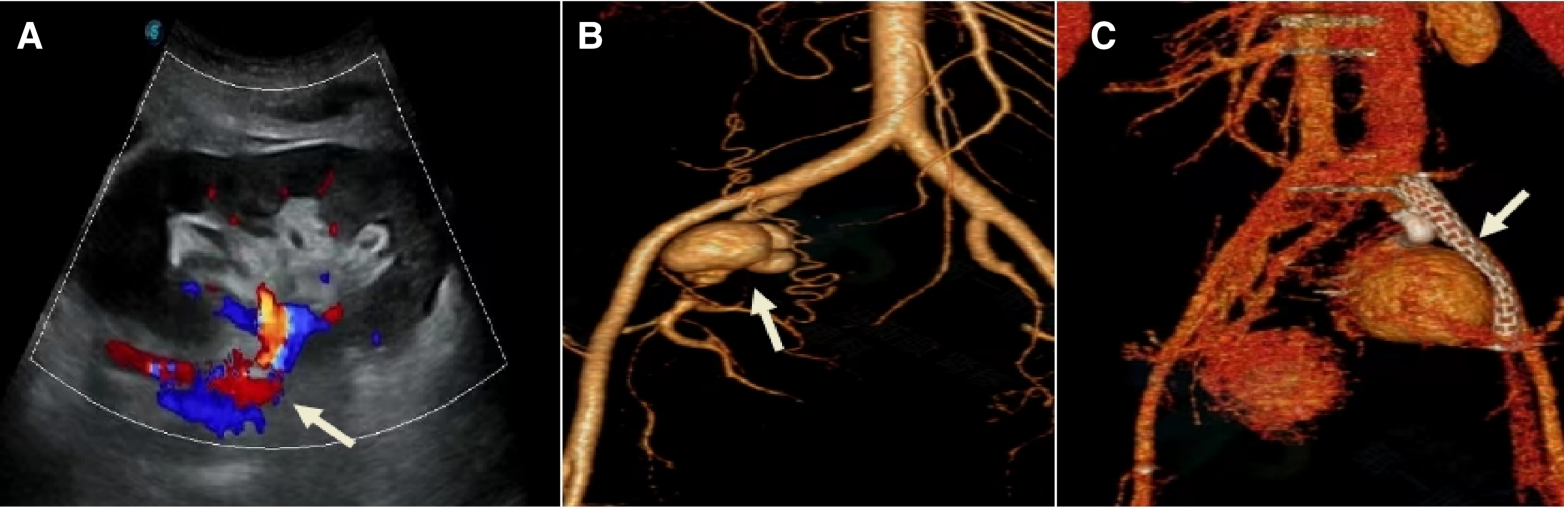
Treatment modality of the transplanted renal aneurysm. Patient 7, female, 34 years old, 2.5 months after renal transplantation, oliguria for 1 day, Scr 354 umol/l. (A) Ultrasound of the transplanted renal before admission: Poor perfusion of the renal, with an anechoic area behind the right external iliac artery, about 32 mm × 36 mm in size, connected to the renal artery, with a channel opening of about 6 mm in width. (B) DSA: Multiple aneurysmal protrusions were seen in the transplanted renal artery. (C) One Viabahn 187 mm–50 mm covered stent was placed so that its distal end was anchored to the main stem of the graft renal artery and the proximal end was anchored to the proximal end of the right internal skeletal artery after release.
